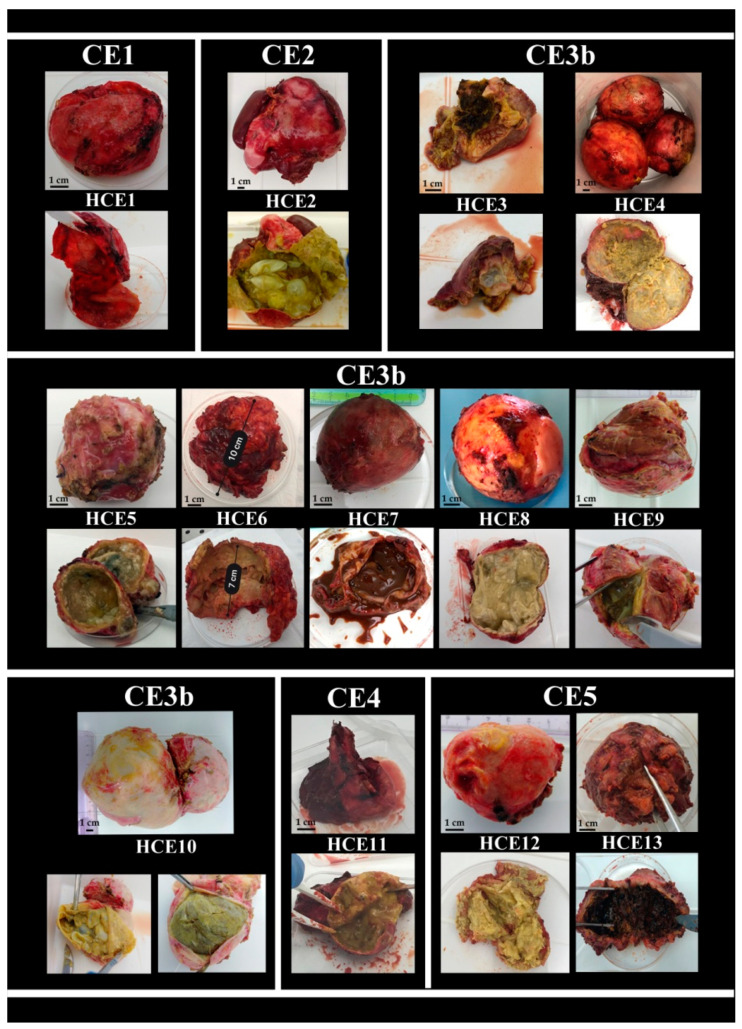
External and internal features of cysts surgically enucleated from 13 patients, displayed according to ultrasound (US) stadiation.
